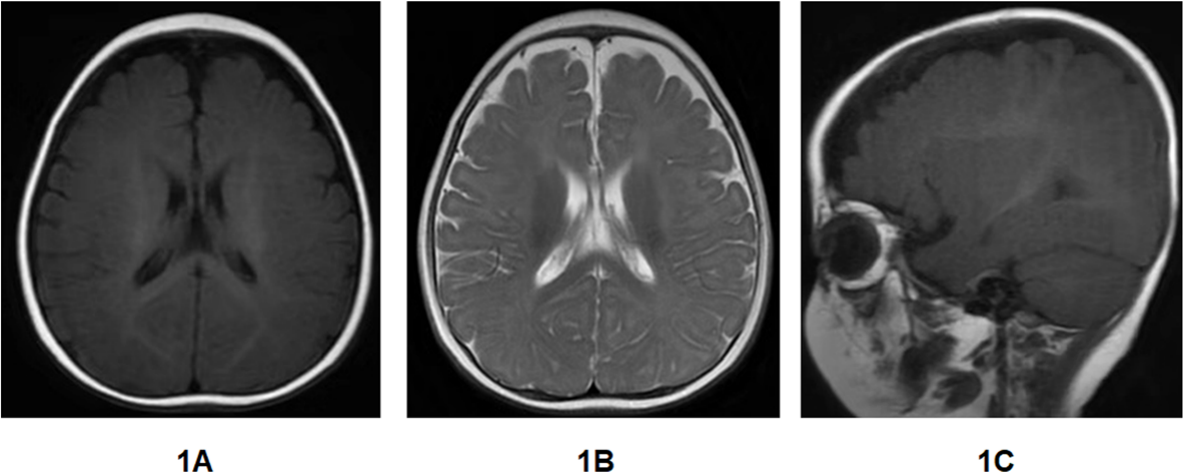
Magnetic resonance imaging of the proband’s brain. 1A Transverse position (T1WI). An increased distance can be seen between the left frontal lobe and the inner plate of the adjacent skull. 1B Transverse position (T2WI). The increased extracerebral space in the left forehead is filled with cerebrospinal fluid, and the local sulcus is widened. 1C Sagittal position (T1WI). Widening of the extracerebral space is seen in the prefrontal lobe
